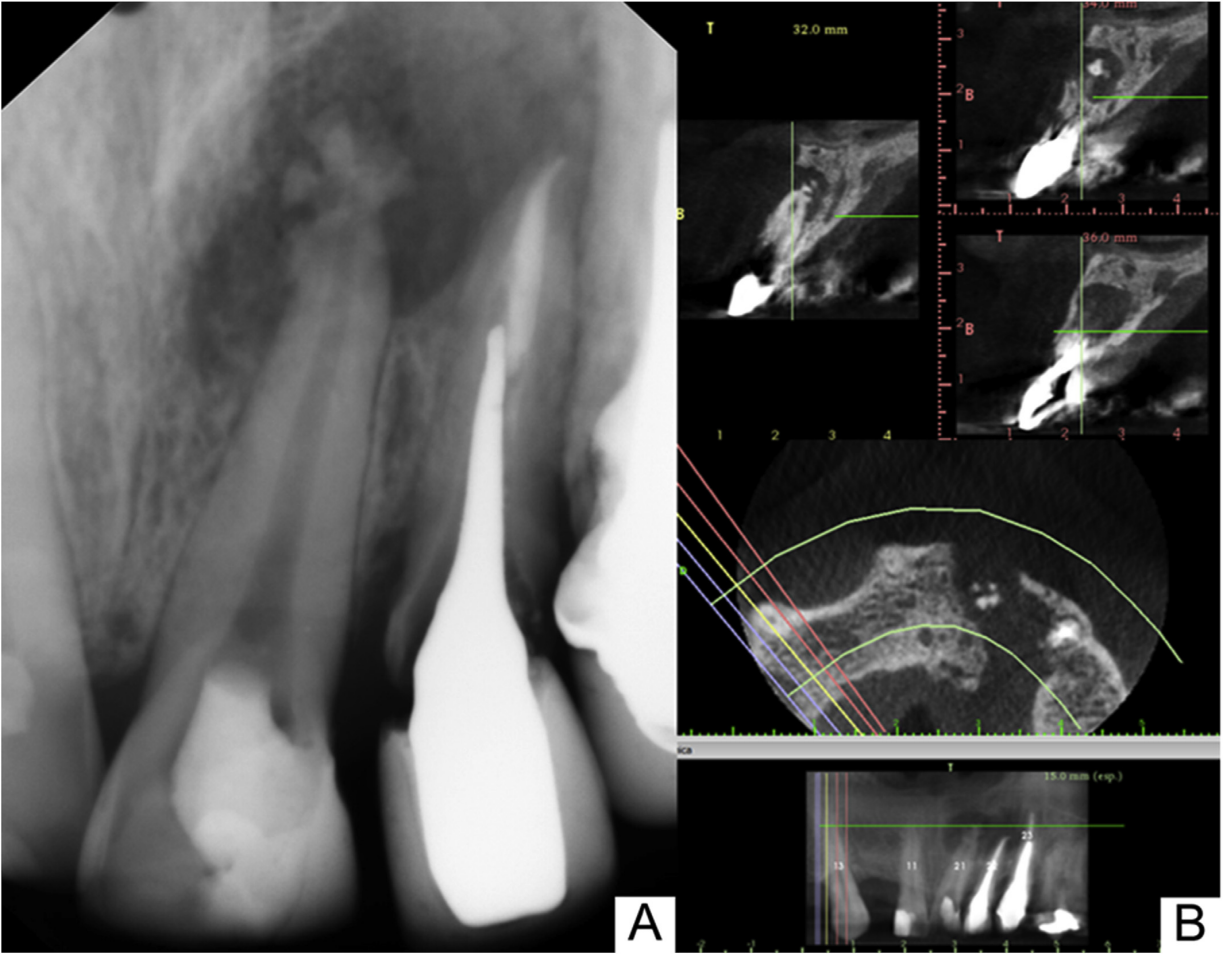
(A) Preoperative radiograph image showing fan-shaped radiopaque image associate with radiolucent area on the apex of the maxillary left central incisor extending apically to the lateral incisor, associated with radiopaque areas. (B) CBCT image showing hypodense, heterogeneous, irregular edges.
